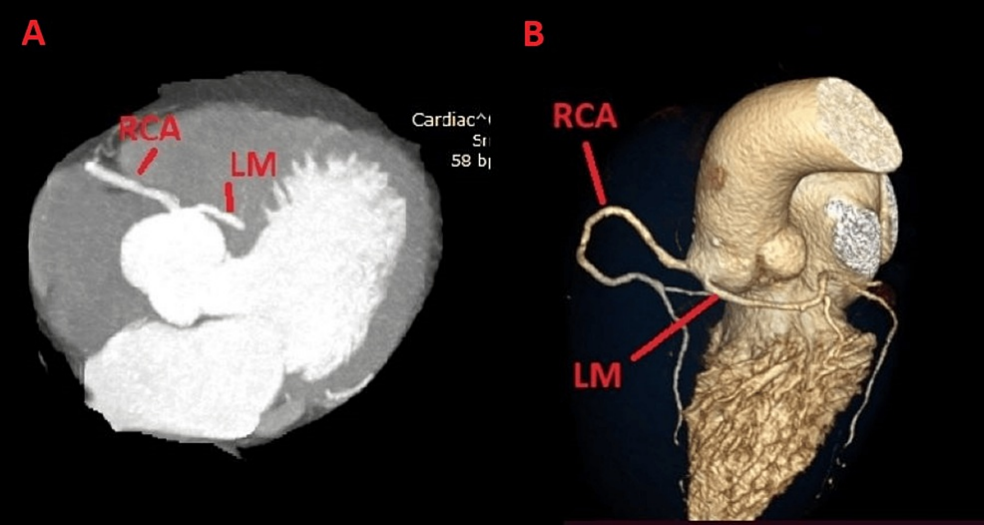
Case 1 A) Two-dimensional coronary CTA image showing LM originating from the right coronary sinus with a distinct ostium from RCA. B) Three-dimensional coronary CTA image showing LM originating from the right coronary sinus with a distinct ostium from RCA with pre-aortic course. CCTA: Coronary CT Angiogram; RCA: Right Coronary Artery; LM: Left Main
